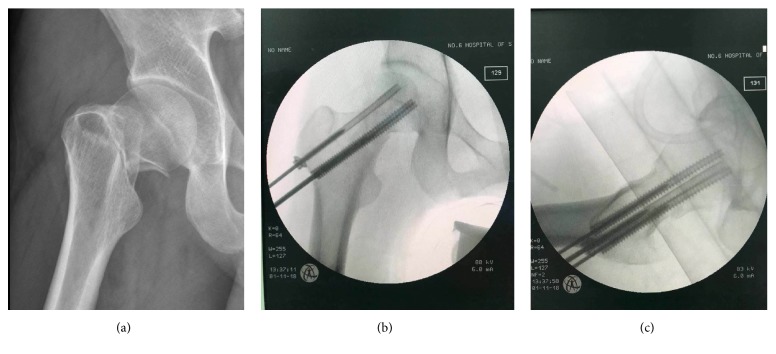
Clinical example: a 29-year-old male patient suffered a left FNF with disrupted posteromedial cortex after a motor vehicle collision. (a) The anteroposterior plain radiograph of pre-operation. (b-c) The intraoperative view after closed reduction and fixation.
